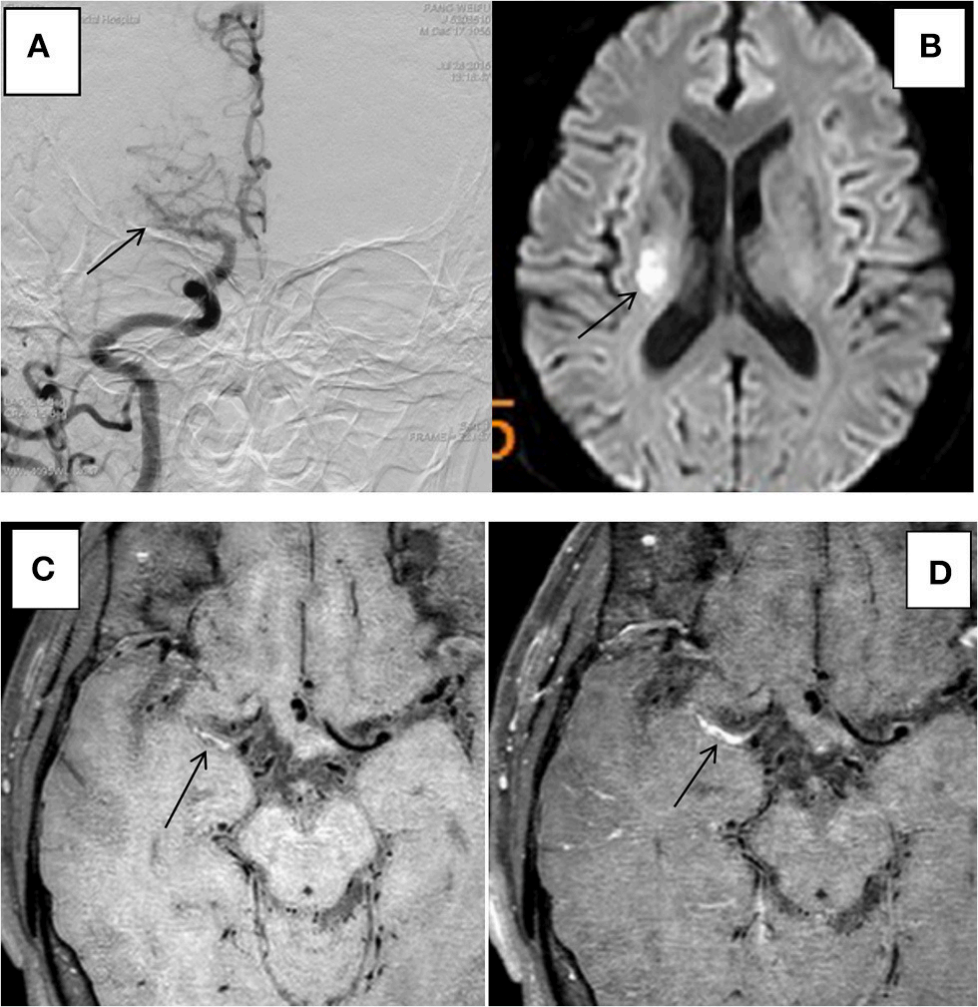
HR-MRI of a culprit MCA occlusion in an 59-years-oldmale. (A) DSA showed occlusion of the right MCA. (B) DWI showed that the right half of the oval center was limited by diffusion. (C) HR-MRI Tl WI showed plaque formation in the Ml segment of MCA. (D) High-resolution enhanced Tl WI showed marked enhancement of the Ml segment of MCA.
